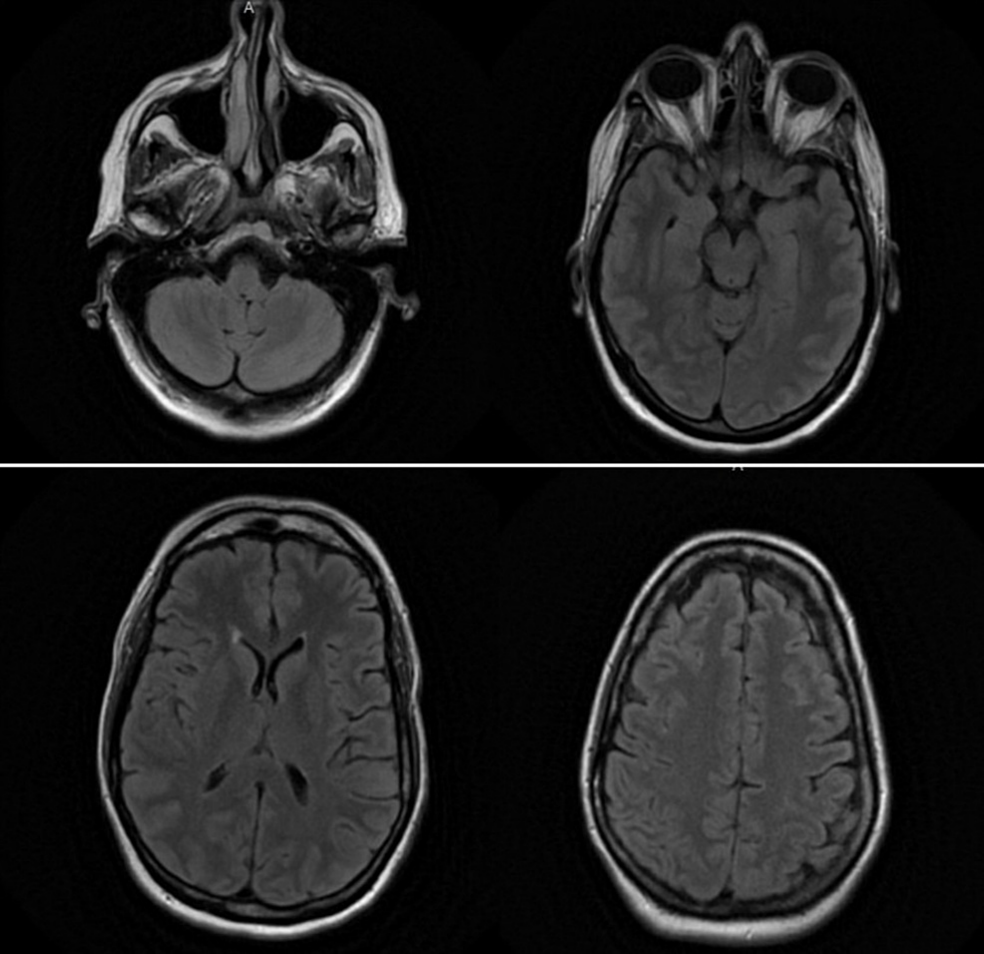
Axial FLAIR MRI demonstrating normal findings. FLAIR: Fluid-attenuated inversion recovery.
